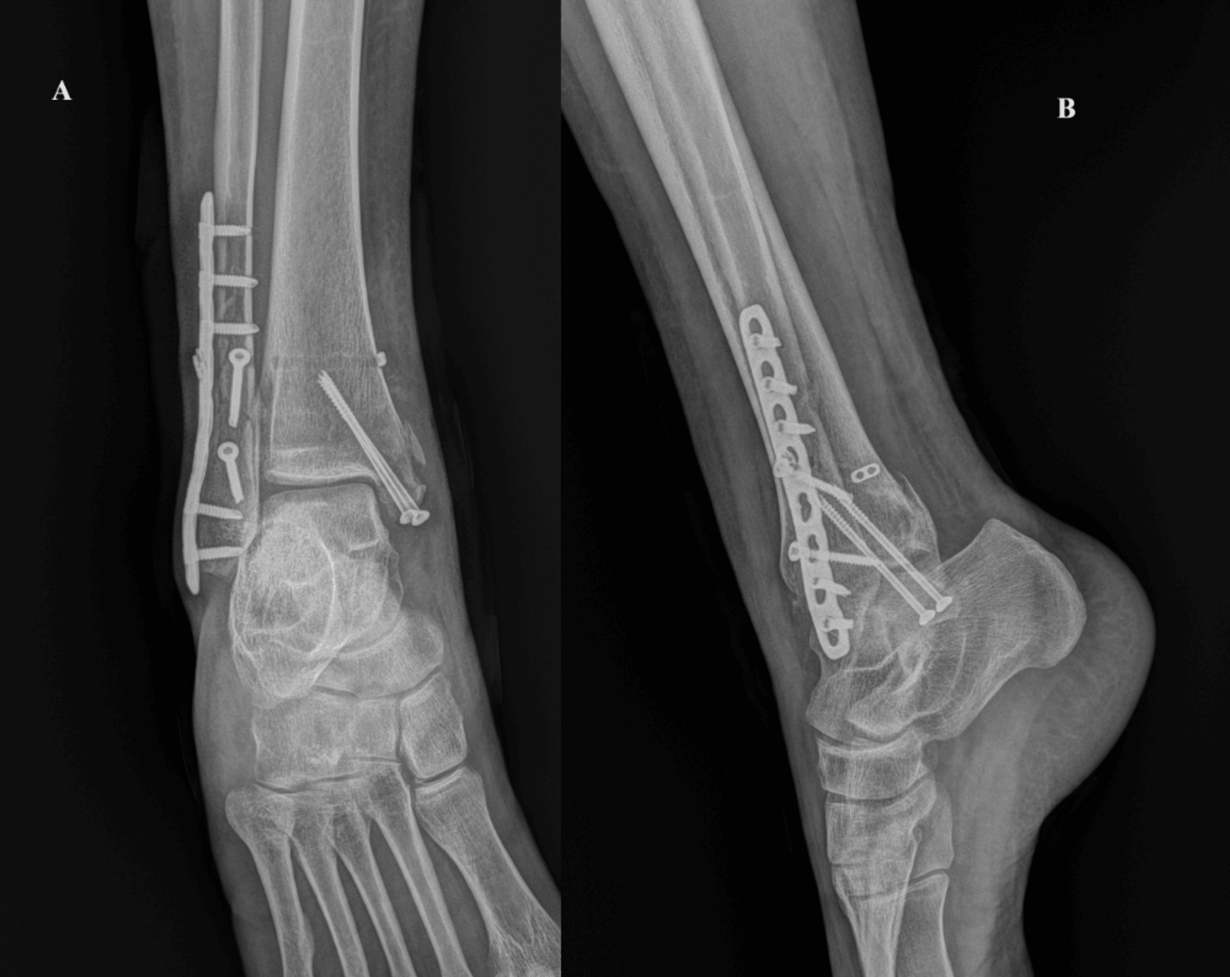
A radiography of the right ankle in anteroposterior (A) and lateral (B) views after fracture surgery This image shows fixed fractures of the malleolus with plate and screws
